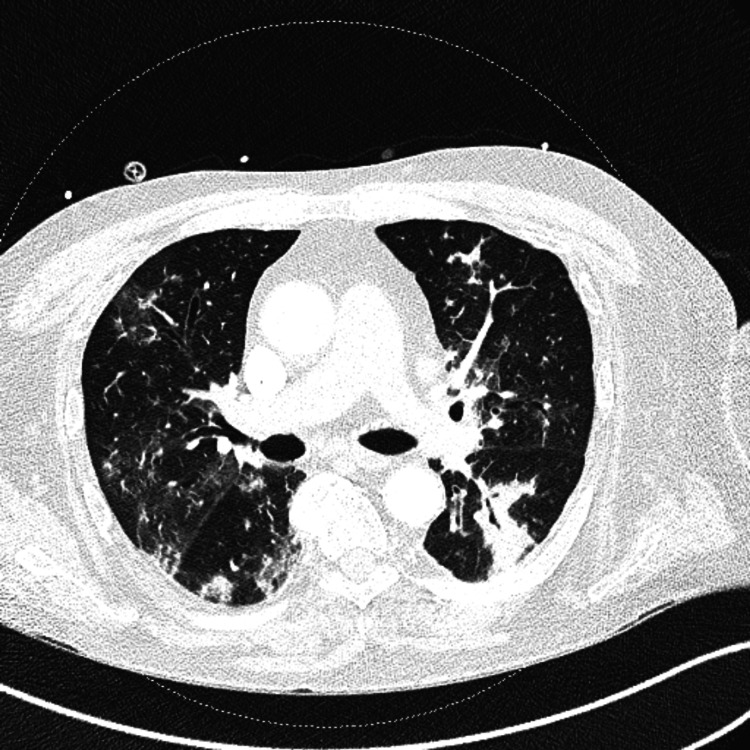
CT pulmonary angiogram showing florid patchy peri-bronchial opacification CT: computed tomography
